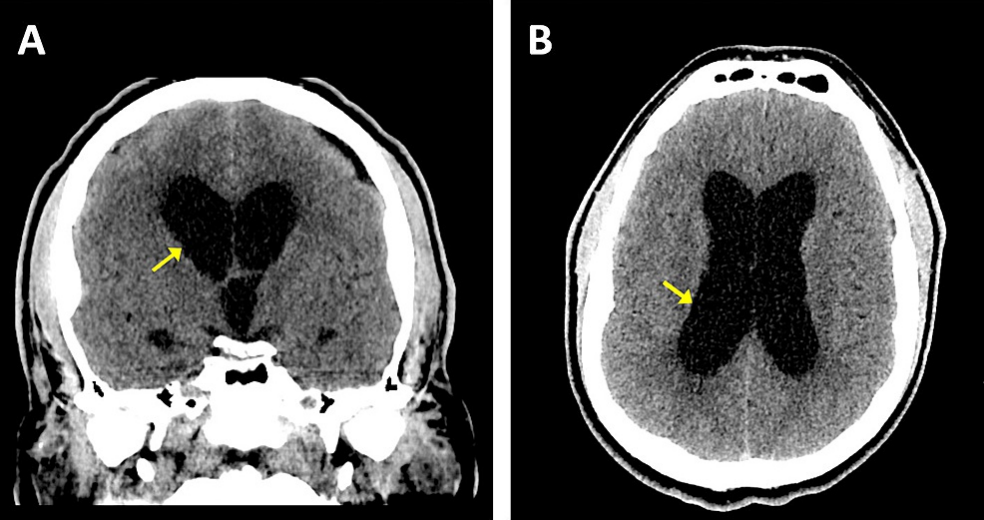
Preoperative CT findings revealing significant obstructive hydrocephalus shown by arrows in the coronal plane (A) and axial plane (B).
